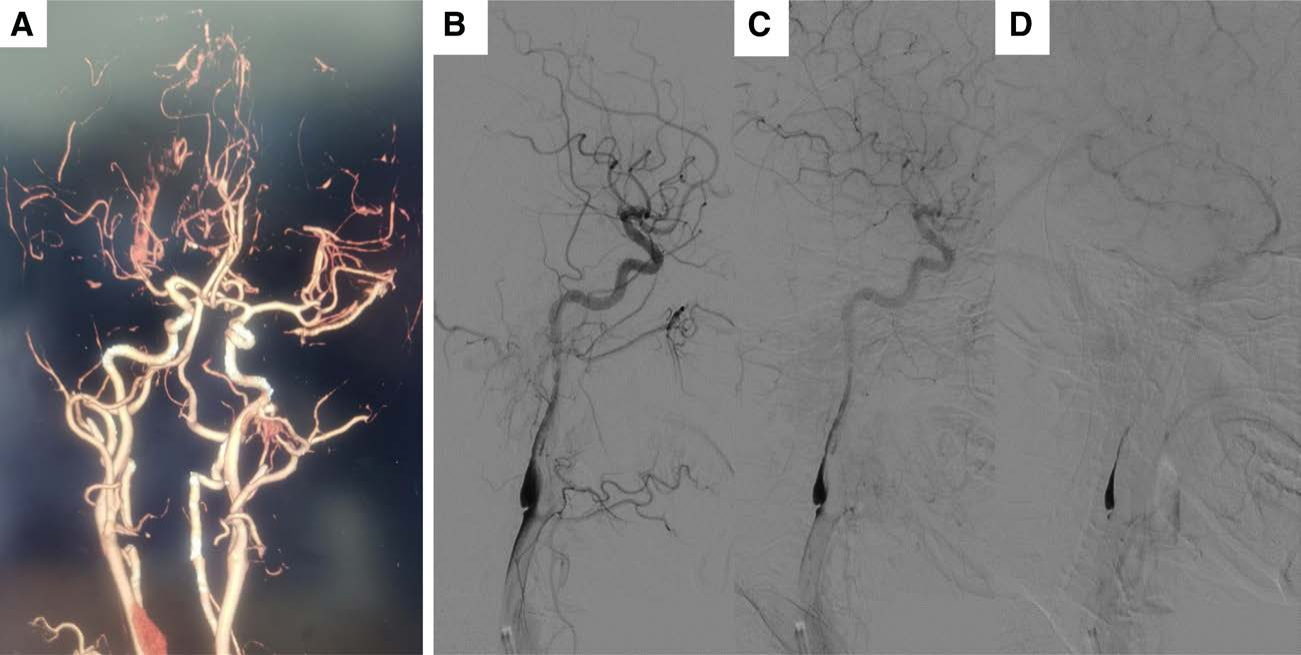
(A) Computed tomography angiography confirmed occlusion of the right middle cerebral artery. (B–D) DSA showed a shelf-like filling defect of the right internal carotid artery with distal contrast stasis. DSA = digital subtraction angiography.
